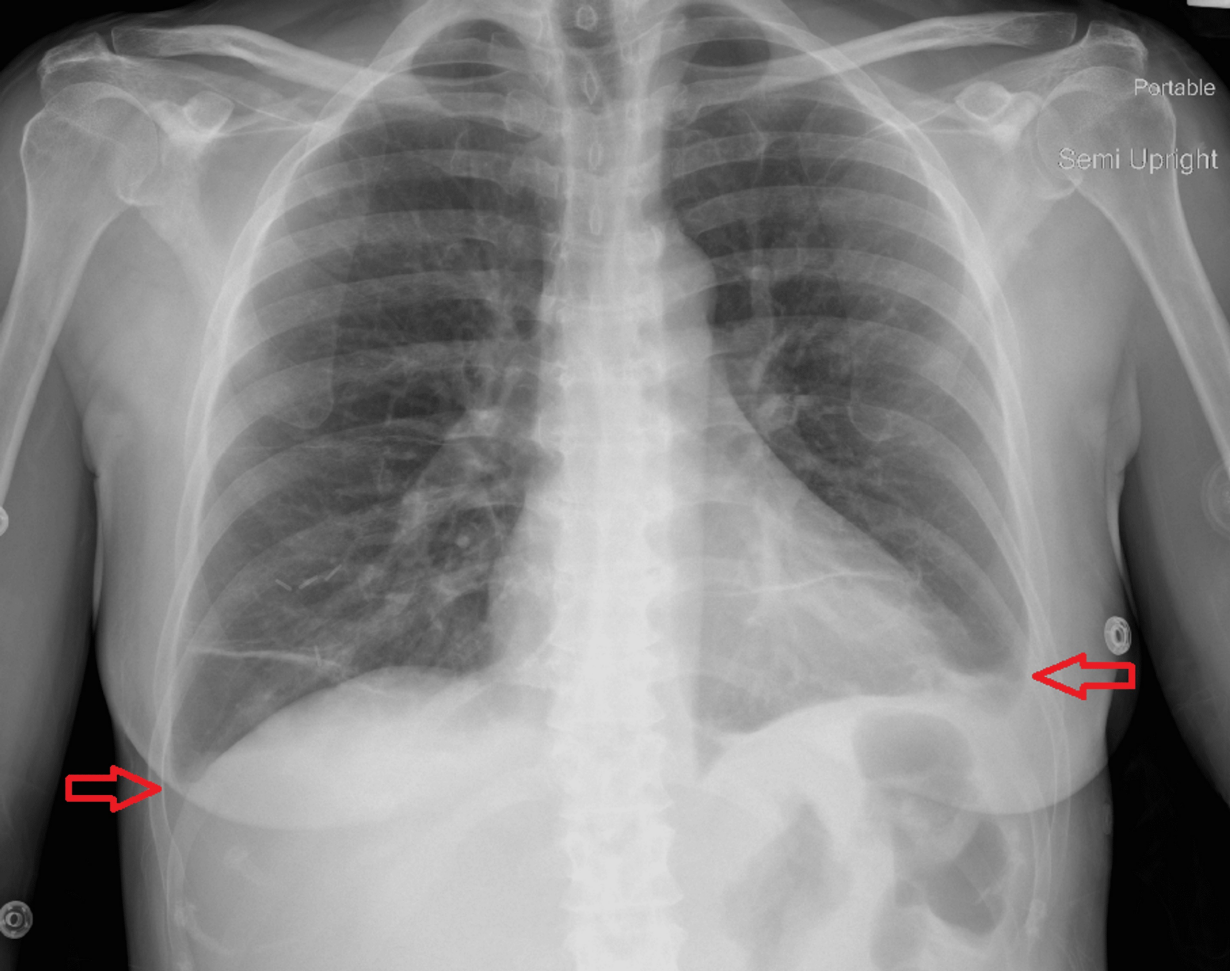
Chest X-ray anteroposterior view showing interval decrease in bilateral pleural effusions on the day of discharge - Day 6 (shown by red arrows)
